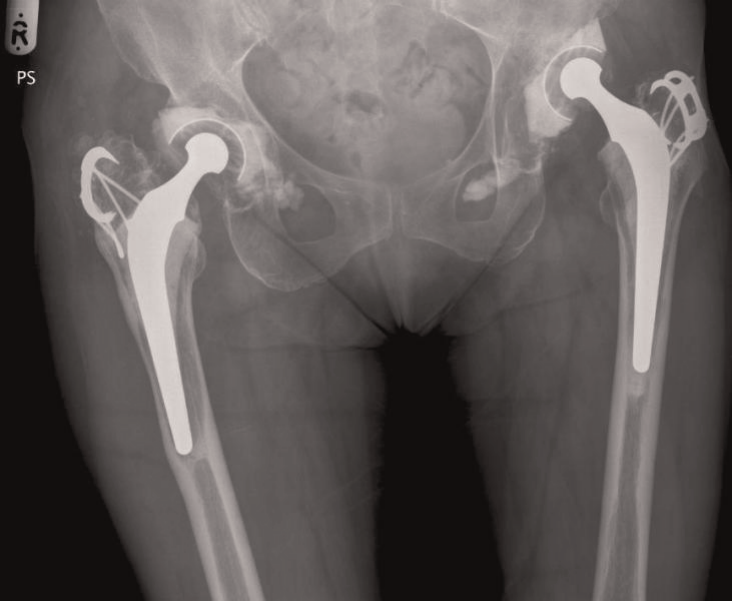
Pre operative radiograph showing loosening of left charnley total hip replacement, performed 20 years ago.
